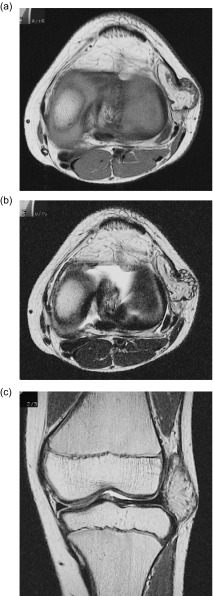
MRI of the tumor demonstrated a high signal intensity area on the T1-weighted image in the axial (a) and T2-weighted image in the axial and coronal views (b, c), and heterogeneously, a low signal intensity area was seen in the tumor. The tumor was located in the extra-capsular lesion of the lateral knee joint and had a diameter of 4 cm.
